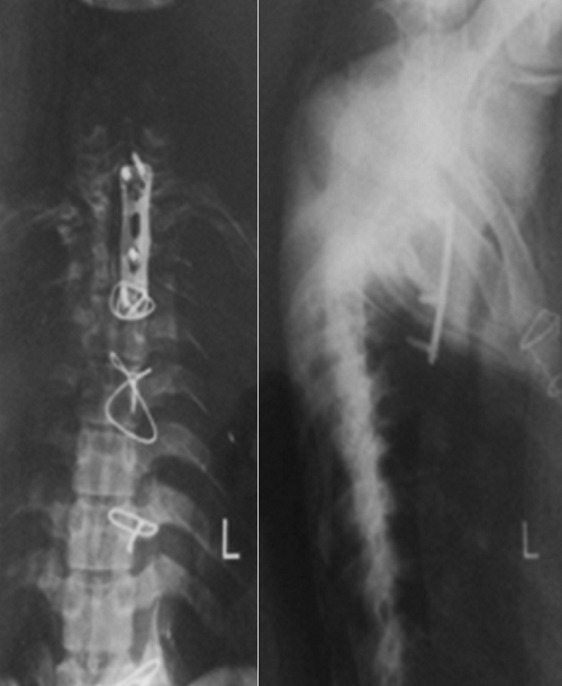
Anterior-posterior + lateral X-rays views
